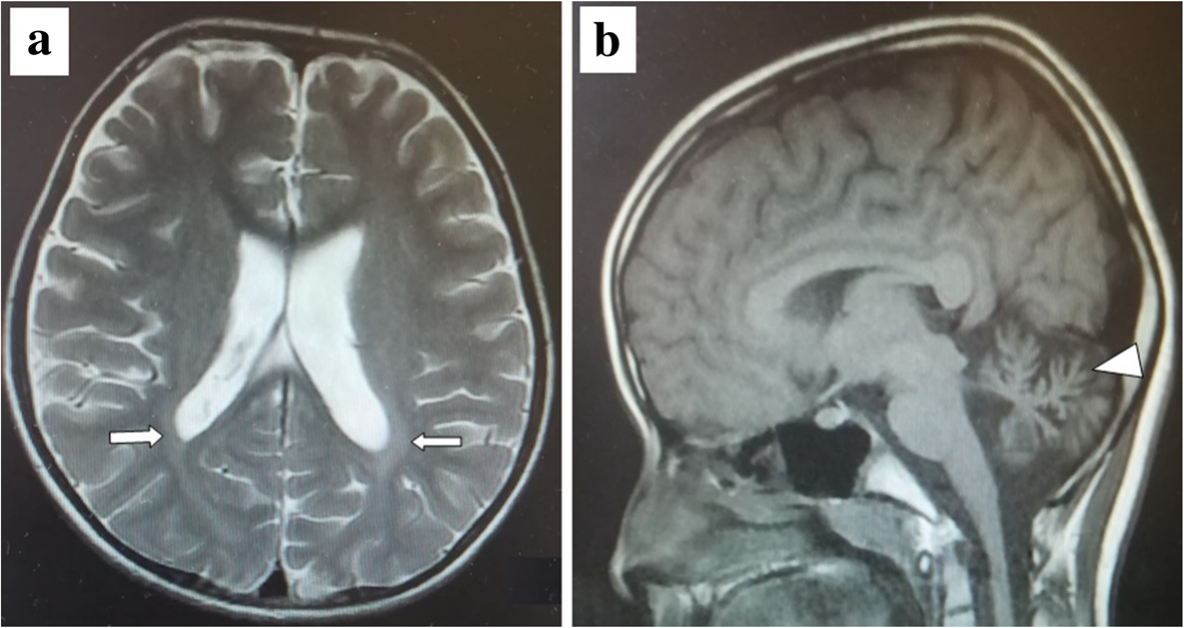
The brain MRI images. a T2-weighted brain MRI image shows high signals adjacent to the bilateral posterior horns of the lateral ventricles (arrows). b T1-weighted brain MRI image shows broadened cerebellar fissures (arrowhead)
